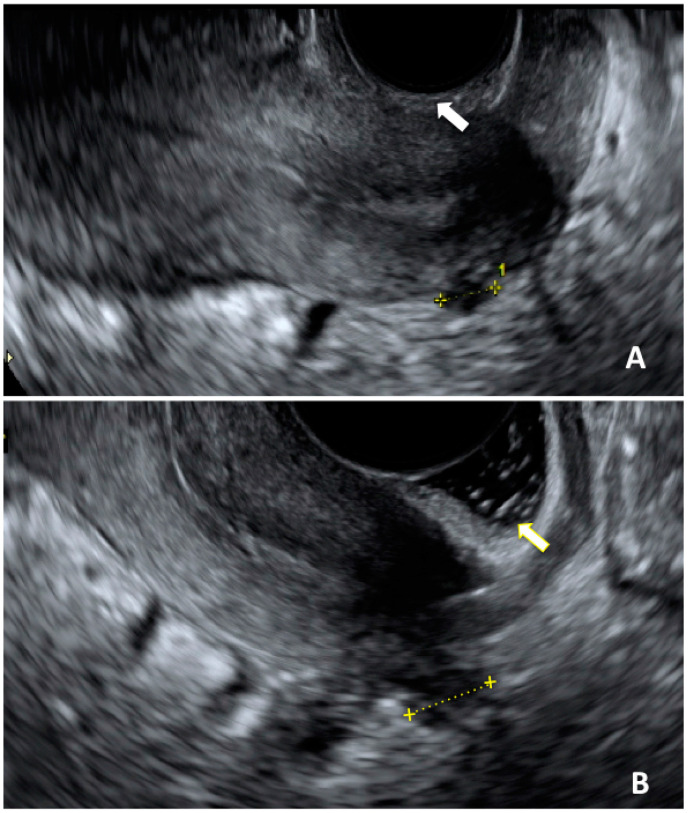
Ultrasonographic image of a DIE nodule affecting uterosacral ligament. (A): acquisition of DIE nodule length in a sagittal plane without sonovaginography (white arrow); (B): same acquisition performed with sonovaginography (yellow arrow).
